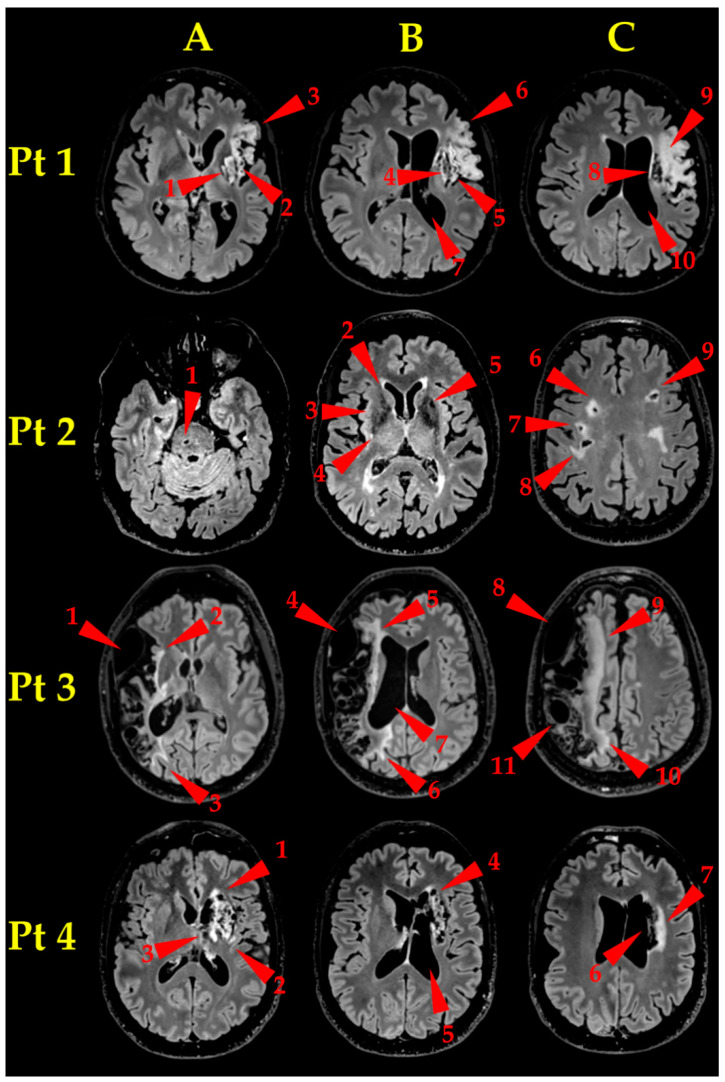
Lesion Morphology before HBOT. FLAIR T2-weighted images. Three axial slices at different levels (A, B, C) are shown for each Pt. Red arrowheads point to the lesions. Pt 1: capsulo-lenticular cavitation (A, B, arrowhead 1 and 4, respectively), insular degeneration (A, B, arrowheads 2 and 5, respectively), *corona radiata* cavitation (C, arrowhead 8), malacic *frontal operculum* (A, B, arrowheads 3 and 6, respectively) and fronto-lateral cortex (C, arrowhead 9), ex-vacuum enlargement of the adjacent segment of left lateral ventricle (B, C, arrowheads 7 and 10, respectively). Pt2: gliotic areas in subcortical and para-ventricular deep white substance, bilaterally, but prevailing on the right side (C, arrowheads 6–9), extending downwards to the basal ganglia (B, arrowheads 2–5) and to the right pons (A, arrowhead 1). Pt 3: wide fronto-parieto-temporal malacic area (whose medial edge is delimited by arrowheads 2–3, 5–6 and 9–10, in A, B and C, respectively). *Ex vacuo* enlargement of right lateral ventricle (B, arrowhead 7). Very extended cyst (A, B, C, arrowheads 1, 4 and 8, respectively) and smaller cyst (C, arrowhead 11). Pt 4: gliotic-malacic area in the left capsulo-lenticular nucleus, partially involving the *insula* (A, arrowhead 1–3), extending to the left caudate nucleus (B, arrowhead 4) and to the ipsilateral corona radiata (C, arrowheads 6, 7). Note also the moderate dilation of the left lateral ventricle (B, arrowhead 5).
